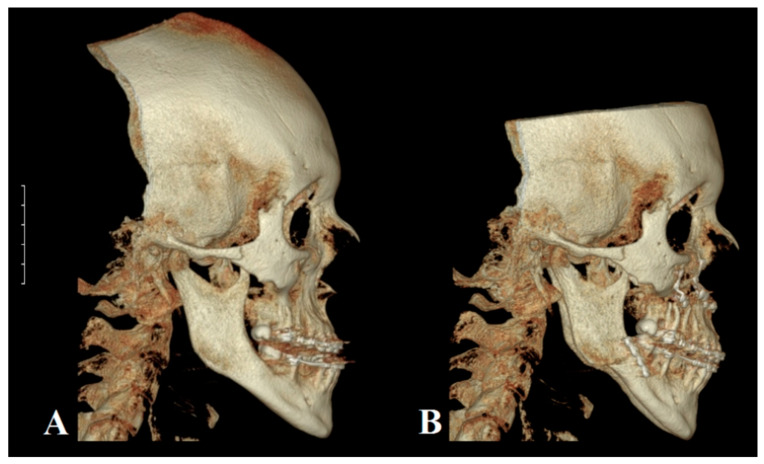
Representative 3D reconstructions of cone beam computed tomography (CBCT) examinations. Patient was diagnosed with class III skeletal malocclusion. (A) CBCT performed during pre-surgical orthodontic treatment. (B) Postsurgical treatment CBCT. The Le Fort I osteotomy of maxilla and the bilateral sagittal split osteotomy of mandible have been performed. The protrusion of maxilla and the retrusion of mandible were conducted to restore proper occlusal status. Osteosynthesis elements are visible (white) within both jaws.
